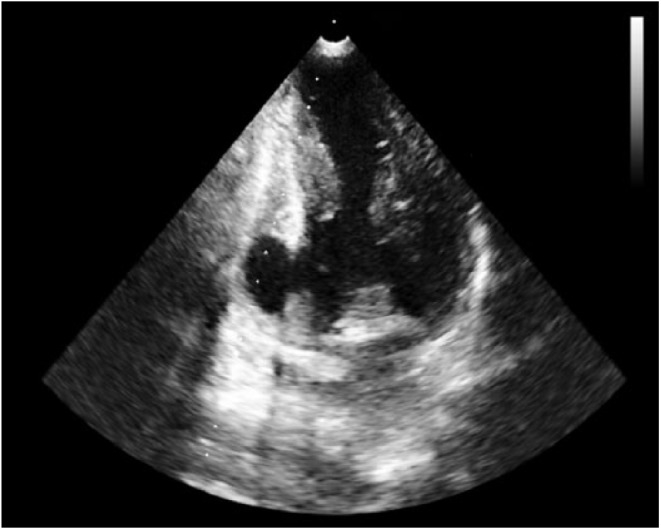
Apical 2-chamber view of transthoracic echocardiogram showing basal inferoseptal and inferior wall akinesis and thinning with pseudoaneurysmal formation (dimensions of pseudoaneurysm were 2.7 × 1.8 cm with neck width 1.6 cm) with dynamic contraction of the remaining walls.
